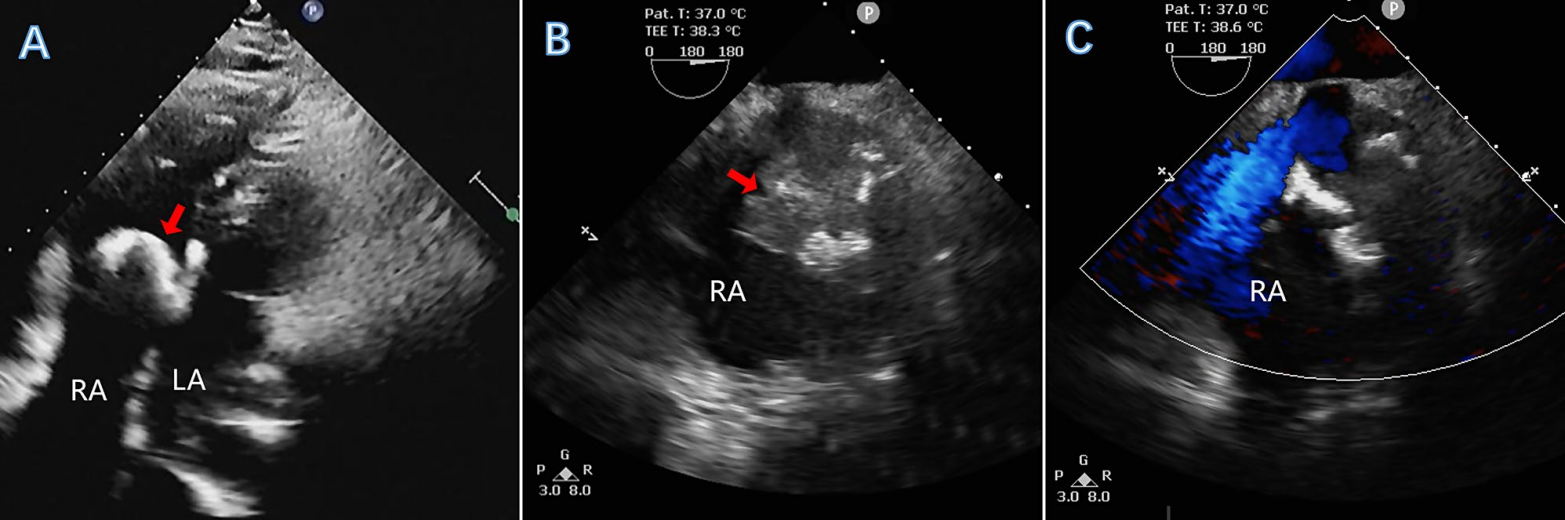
Multiparametric ultrasound images. On the four-chamber view obtained from both TTE and TEE at a 180° angle, a heterogeneous mass was identified in the right atrium, indicated by the red arrow (A and B). Within the mass, an arc-shaped hyperechoic focus was observed, accompanied by broad acoustic shadowing posterior to the mass. Color Doppler imaging revealed no significant blood flow within the mass (C)
